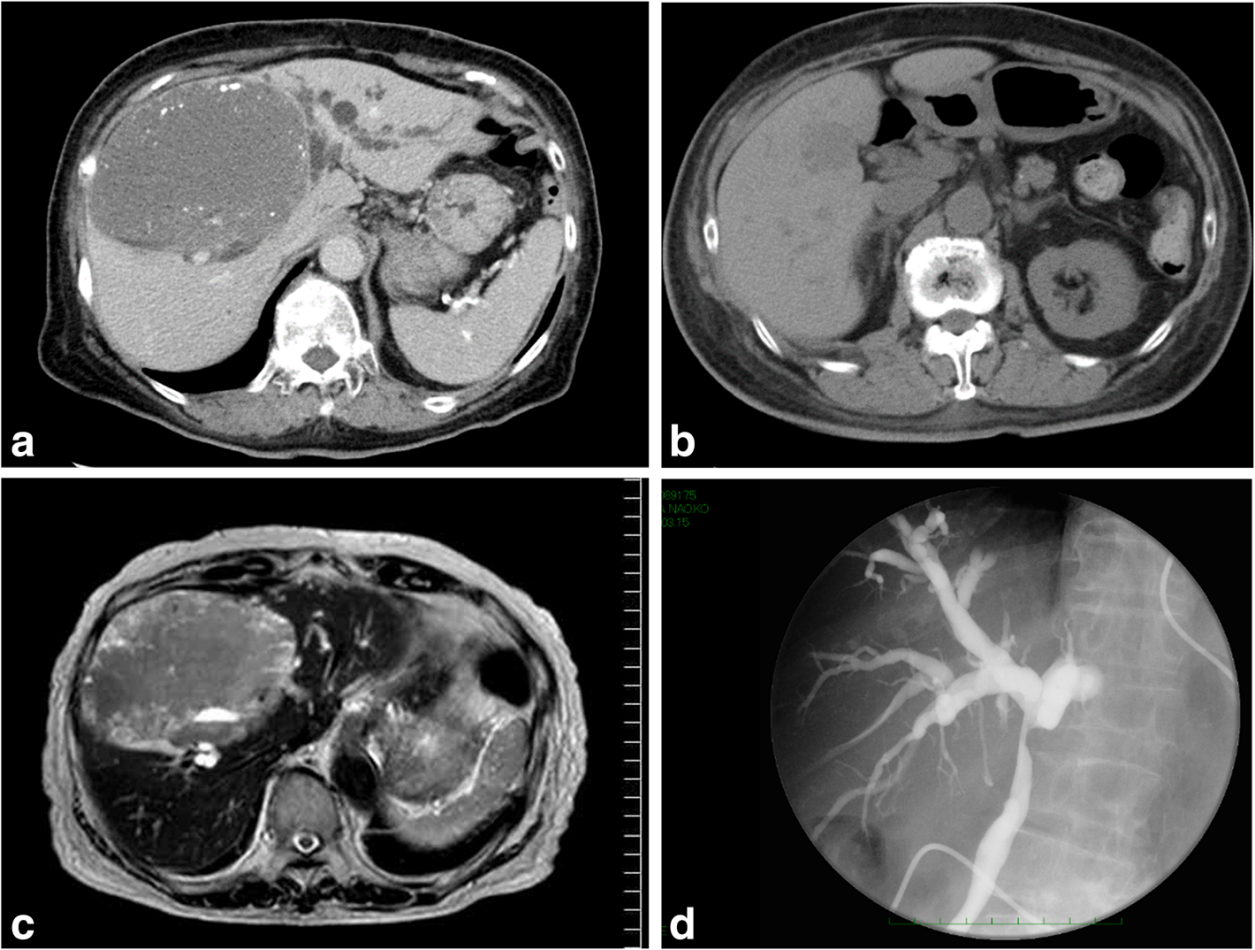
Radiological findings. a Computed tomography (CT) scan of the abdomen showing a well-demarcated liver mass consistent with hemangioma in the anterior segment of the right hepatic lobe to segment IV. The tumor pressed a bile duct in the hepatic portal region, causing an intrahepatic bile duct to expand. b A CT scan performed 5 years prior to the study shows a low-density tumor with a diameter of 28 mm in the right lobe. c The size of the tumor was shown to be 13 cm in diameter in magnetic resonance imaging, with a clearly demarcated border and a heterogeneous signal on T2-weighted images. The tumor had a fluid-fluid level at the tumor margins. d A stricture of approximately 2 cm in length in the common bile duct was observed via endoscopic retrograde cholangiography and suspected to be a result of compression from the outside wall. This caused partial expansion of a right lobe bile duct and high expansion of the left lobe bile duct
